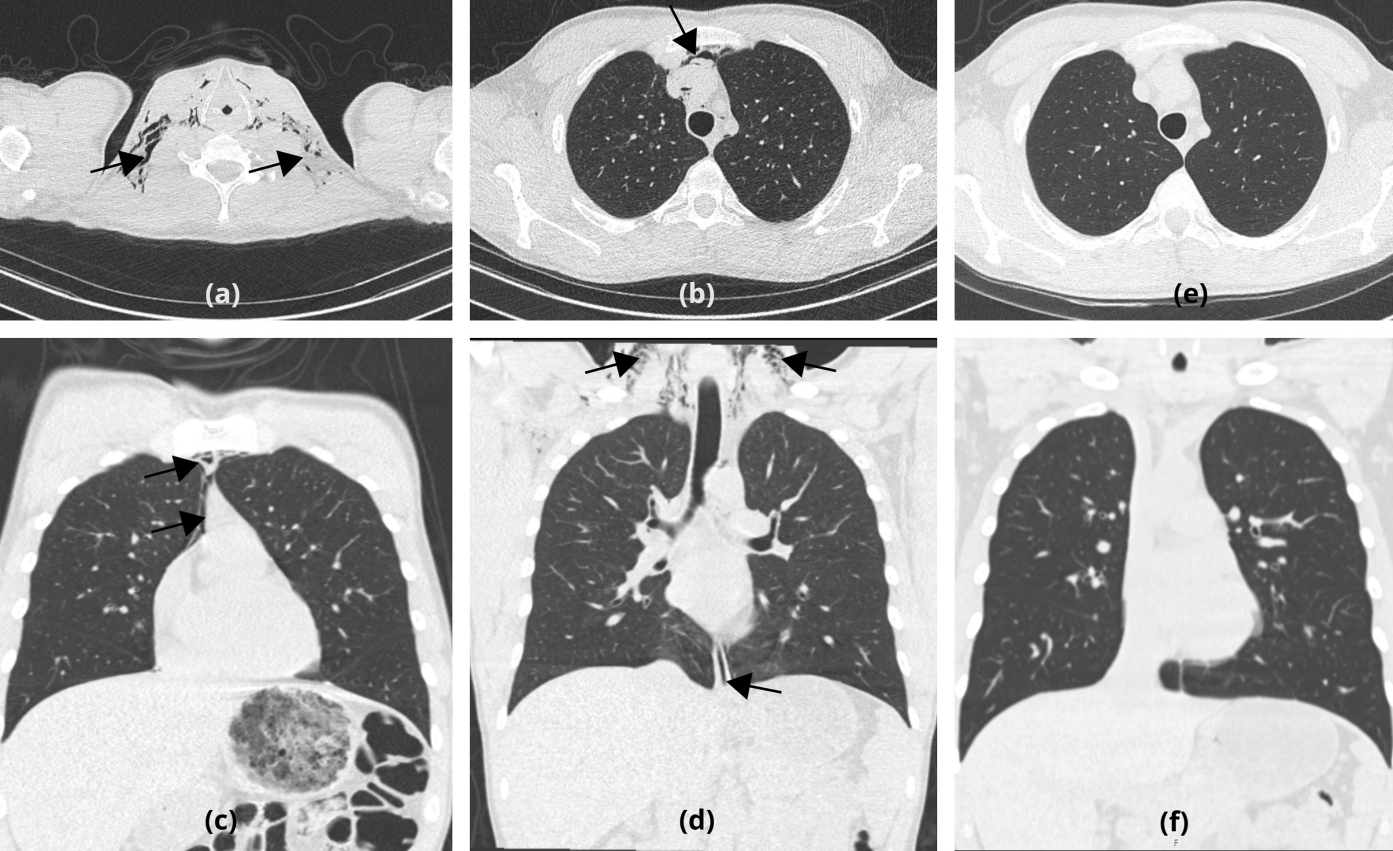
The initial CT images—The axial (a) and the coronal (d) images at the base of the neck showing the subcutaneous emphysema (black arrow), The axial images (b) and the coronal images (c, d) of the thorax showing the thin pneumomediastinum (black arrow). The follow‐up CT images in 4 weeks, demonstrating complete resolution of subcutaneous emphysema and pneumomediastinum (e and f) in both axial and coronal images.
